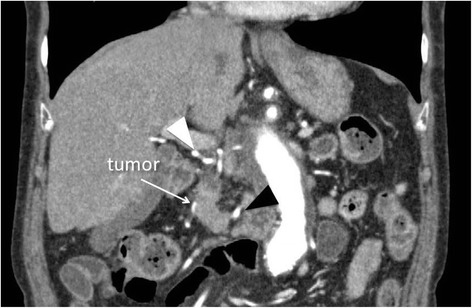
An enhanced CT scan demonstrated there was a tumor (labeled as “tumor”) in the pancreatic head and RHA-PB (*white arrow head*) originating from PIPDA (*black arrow head*) was running through the tumor
